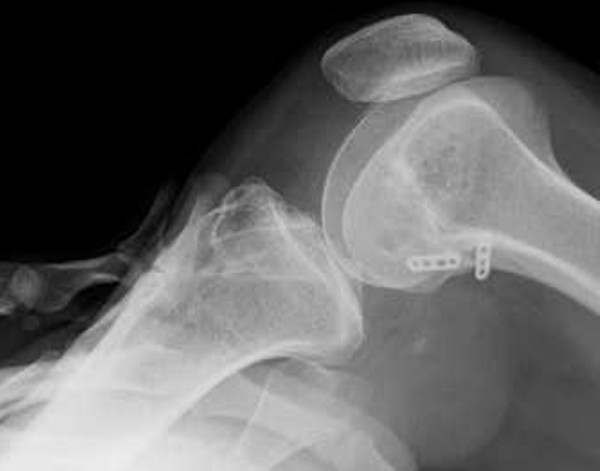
Stress X-ray findings 36 months after ACL reconstruction. The results at 36 months post operative stress radiograph evaluation showed 61% anterior translation of the tibia.
